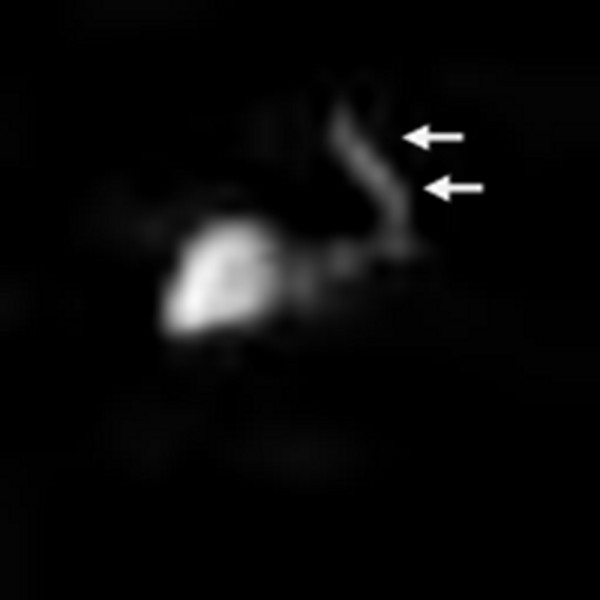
^99m^Tc sulfur colloid scintigraphy (anterior view) showed liver uptake as well as the nodule uptake (arrows) corresponding to pleurocardial splenosis.
